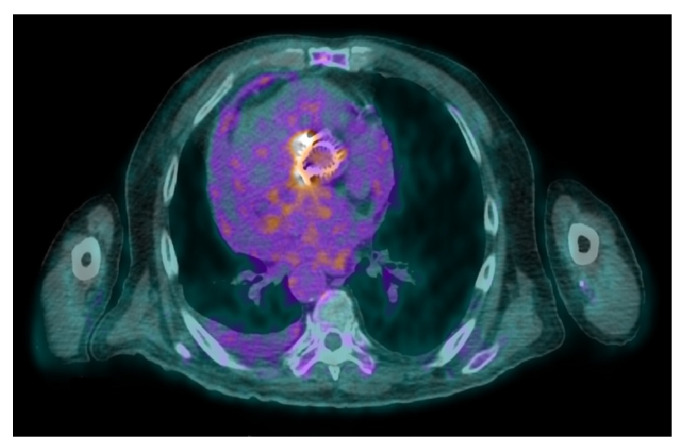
Aortic prosthetic valve infection. 18F-FDG PET/CT fused image demonstrates intense radiotracer uptake at the level of the patient’s aortic valve prosthesis (male, 73 years old).
